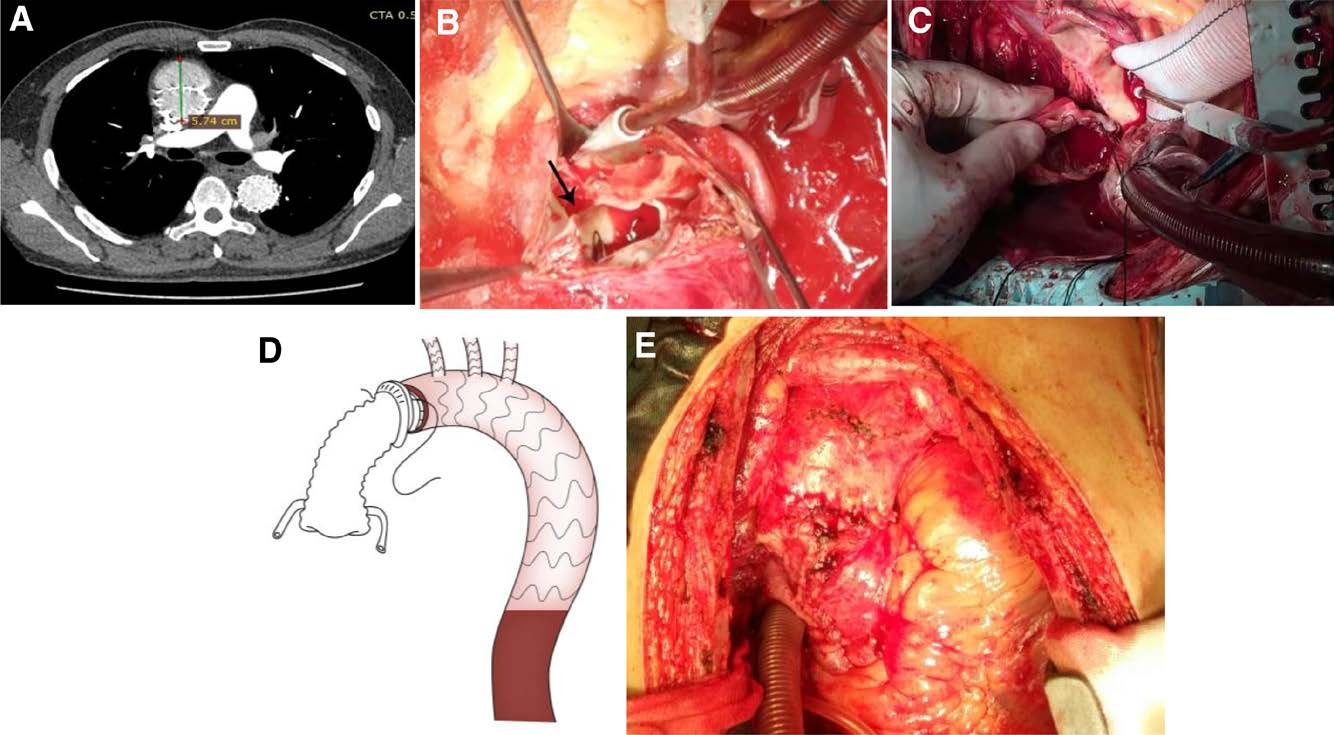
The perioperative images of the second operation. (A) CTA showing a new retrograding dissection starting from the proximal endograft and extending proximally to the aortic root with a maximum diameter of 57.4 mm. (B) Intraoperative image after incision of the ascending aorta. The arrow indicates the entry tear located in the proximal bare spring of the endograft. (C) The steel wires of the bare spring were cut to create an appropriate distal plane. (D) The graft inversion technique of distal anastomosis. (F) The aorta and grafts were wrapped and sutured with pericardium. CTA = computed tomography angiography.
